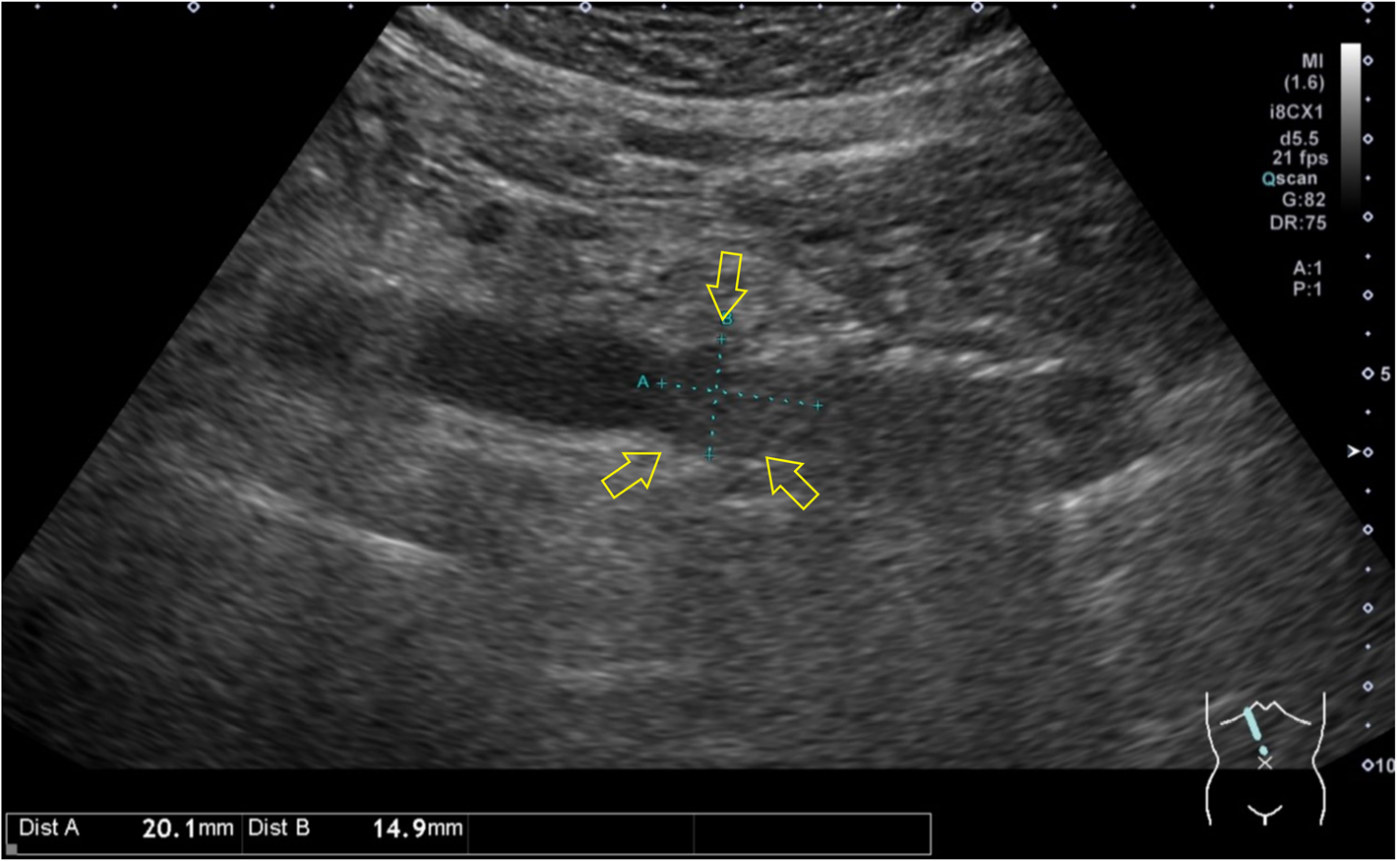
Ultrasonography showed a 2-cm tumor in the distal bile duct
